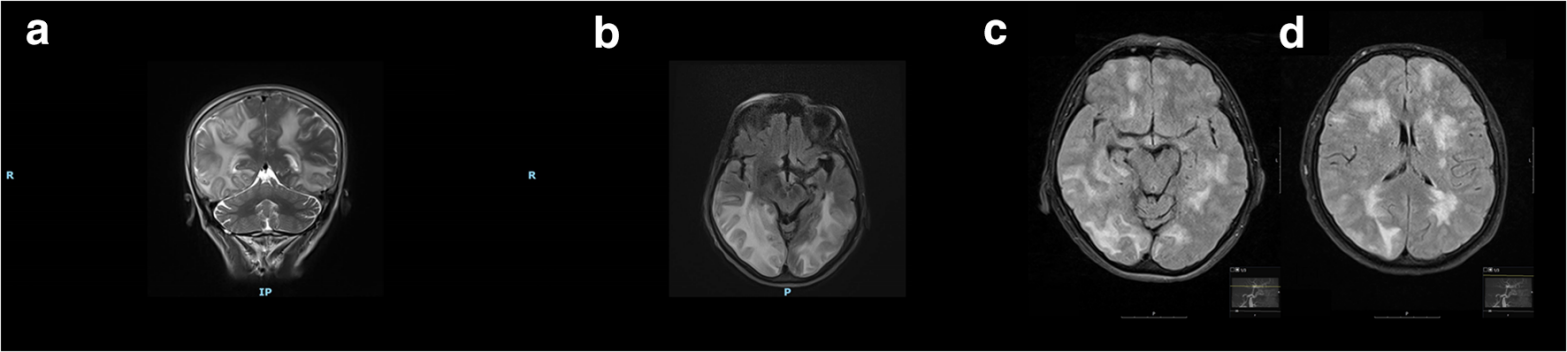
MR imaging of patient 1 with coronal T2 (a) and axial FLAIR (b) images showing the diffuse and symmetrical distribution of vasogenic edema in the cortical and subcortical area of fronto-parieto-occipital lobes. MR imaging of patient 4 with axial FLAIR (c, d) images showing hyperintensity of white matter representing vasogenic edema in the fronto-temporo-parieto-occipital area
